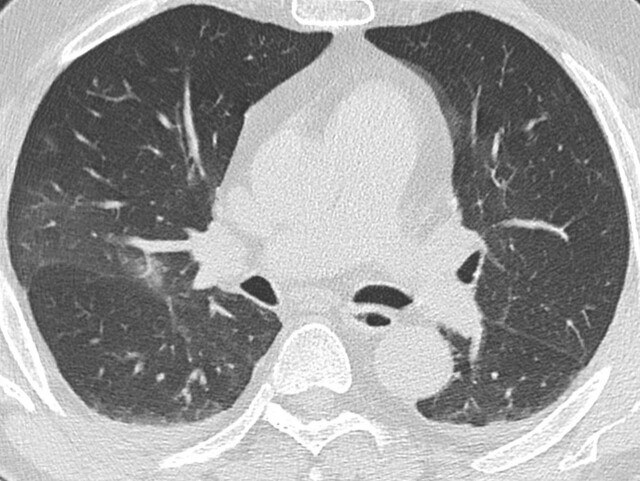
CT at presentation of a 62-year-old female with fever, cough and dyspnoea: perifissural GGOs in posterior segment of right upper lobe and small bilateral pleural effusion. GGO, ground-glass opacity.
